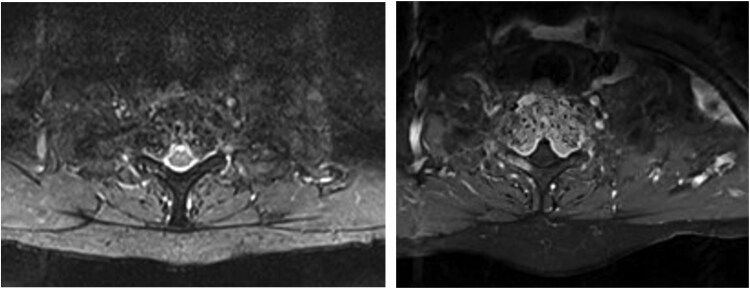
T2W and T1W FS axial MRI images showing changes extending into bilateral pedicles and bulging cortical margins. The vertebral canal is narrowed by the extradural haemangioma component, with distortion but not compression of the spinal cord. Cord signal is normal. There is bilateral compression of the T1 nerve roots.
